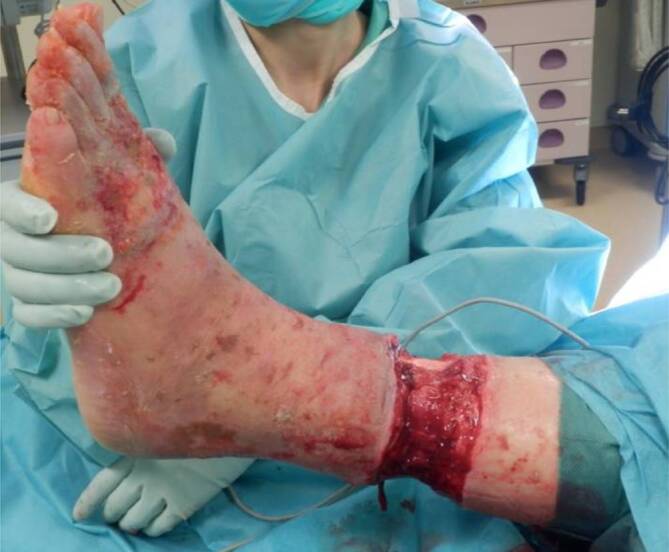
In einer postoperativ durchgeführten CT-Angiographie zur Beurteilung der Durchblutungssituation stellten sich umschriebene „hard plaques“ in der A. poplitea mit ca. 50 % Durchmesserstenose, eine kräftige Kontrastierung des Truncus tibiofibularis sowie der A. tibialis anterior und fibularis beidseits dar. Die A. tibialis posterior war bis zum Übergang auf die Fußarkade etwas kaliberschwächer.
